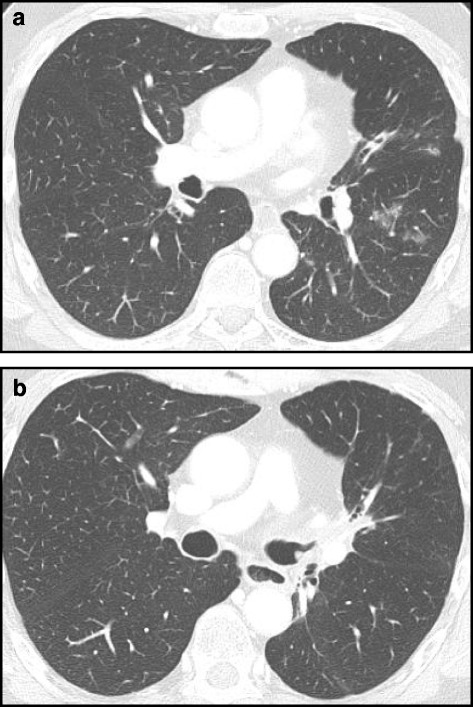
(a) CT scan of a patient in treatment with Sorafenib showed, in the left lung, multiple ground glass nodules in the posterior segment of superior lobe. (b) CT image in the same patient after 4-week withdrawal from chemotherapeutic agent, demonstrating the disappearance of the nodules
